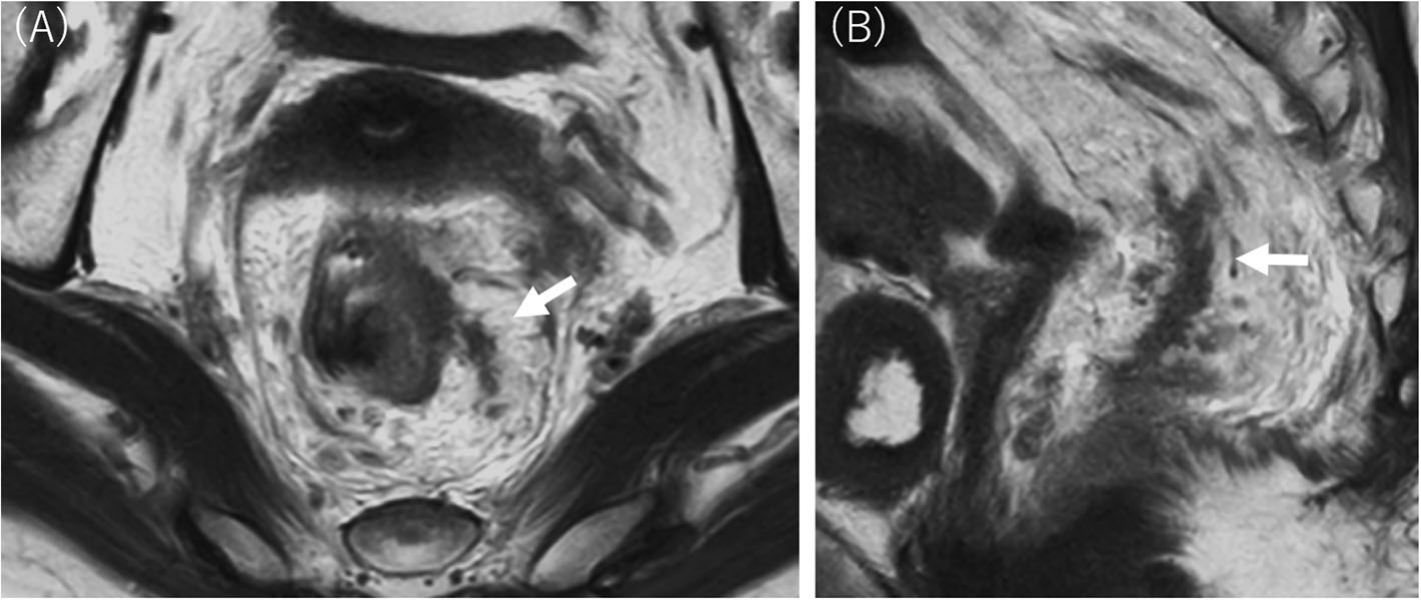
Axial (a) and sagittal (b) T2-weighted magnetic resonance images. The tumor signal extended into the vascularis outside the muscularis propria of the bowel wall (arrows). This case was scored as a 4
